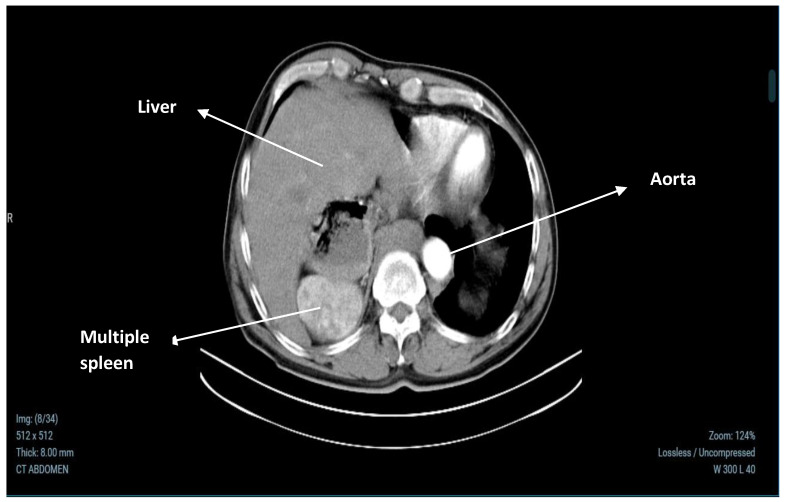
Contrast-enhanced CT scan demonstrating multiple accessory spleens situated in the region of the right adrenal gland. These accessory spleens can make it challenging to accurately localize and characterize adrenal lesions, as their contrast enhancement characteristics may closely resemble those of adrenal masses.
